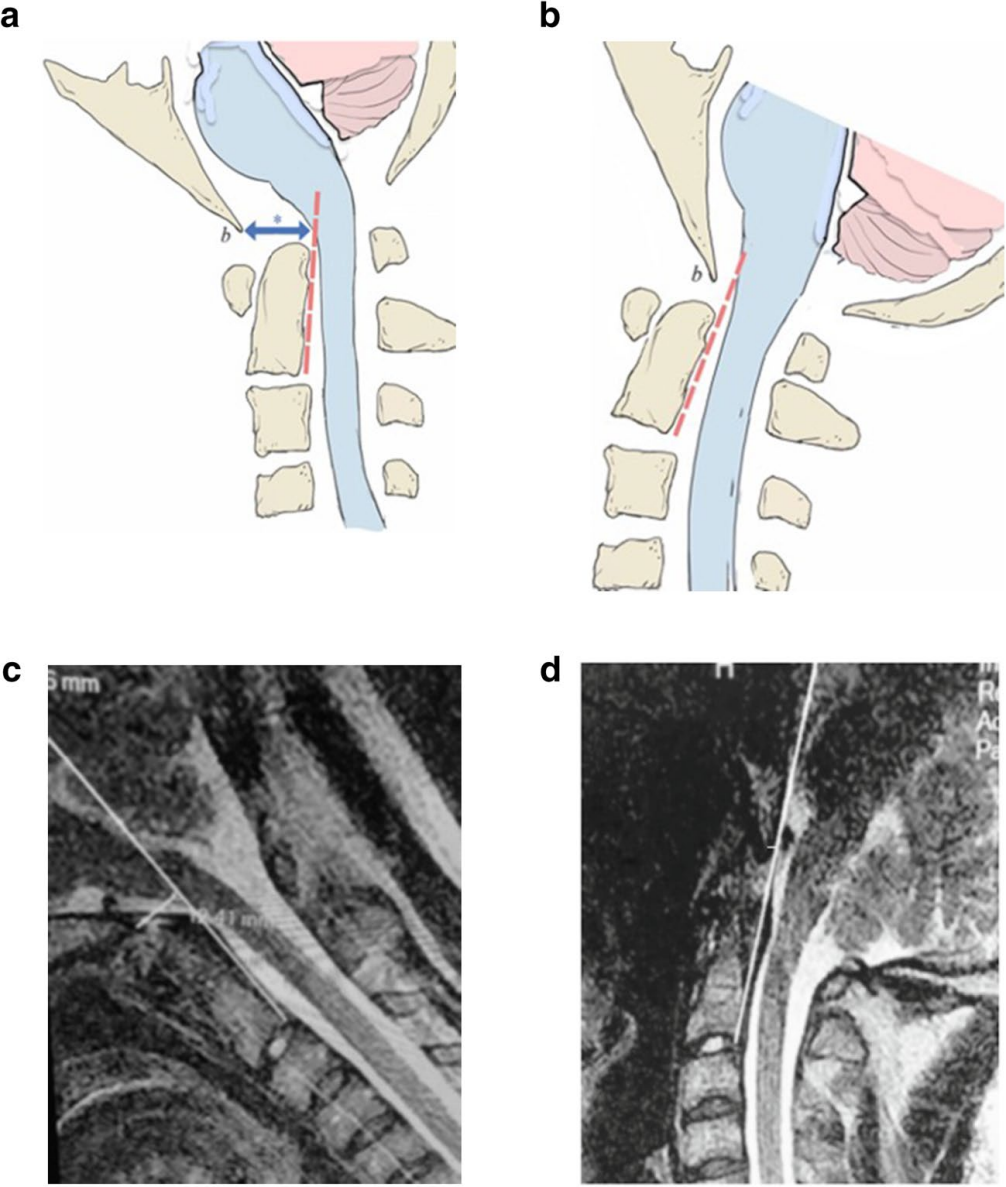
a Pathological translation of the basion with respect to the odontoid. Normally, between flexion and extension, the basion (b) pivots over the odontoid with < 2 mm of translation. In Fig. 2a, the cervical spine is in flexion, and the basion has translated anteriorly causing a bend in the brainstem. Note the BAI (*)—the interval measured from the basion to the posterior axial line (dashed line) is greater than the width of the spinal cord. b The cervical spine in extension shows straightening of the brainstem and a upper spinal cord and a shortened BAI. The change in BAI represents a pathological translation of the basion with respect to the spine. c MRI, upright, (dynamic), mid-sagittal, flexion view, T2 weighted (0.6 Tesla, Fonar Corp). The basion axis interval is 12 mm. d MRI, upright, (dynamic), mid-sagittal, extension view, T2 weighted (0.6 Tesla, Fonar Corp). The basion axis interval is 5 mm. Therefore, the BAI in flexion (12 mm) minus the BAI in extension (5 mm) represents a pathological translation of 7 mm
